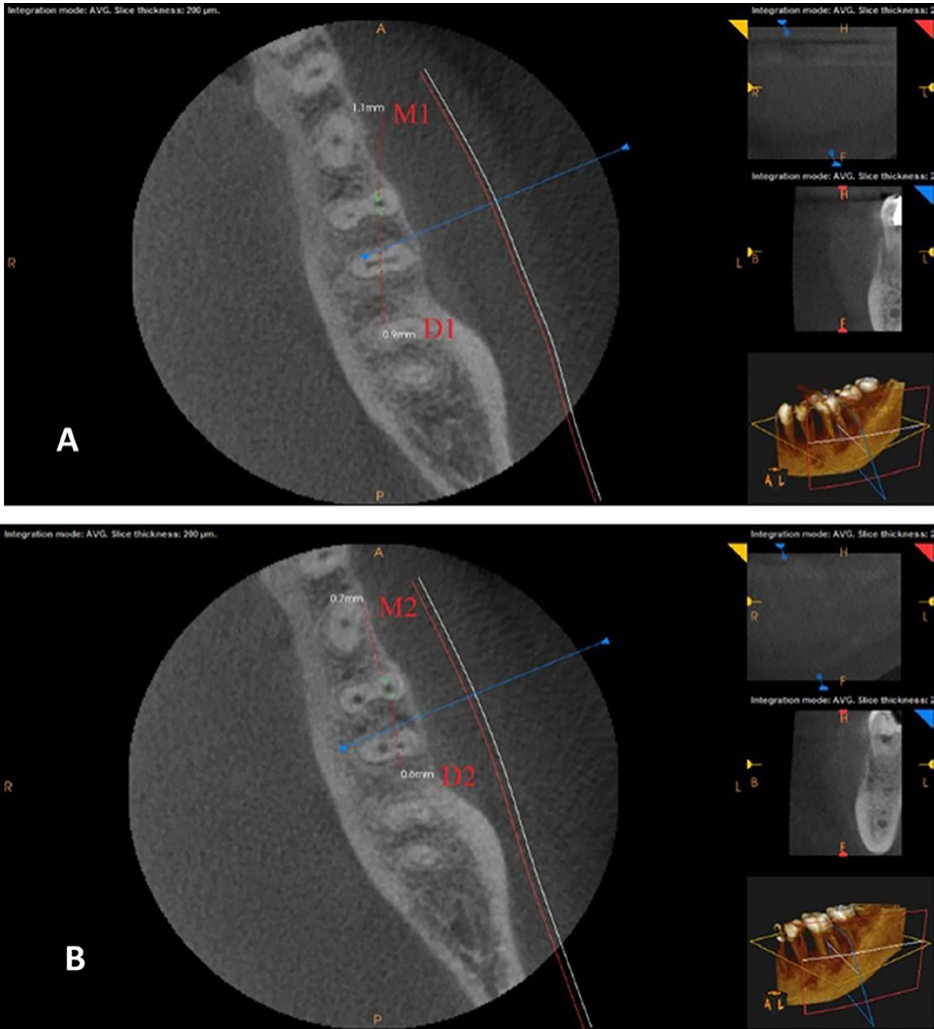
Points of measurements used for determining canal shaping in MB canal for pre-instrumentation (A) and post-instrumentation (B) CBCT images.
